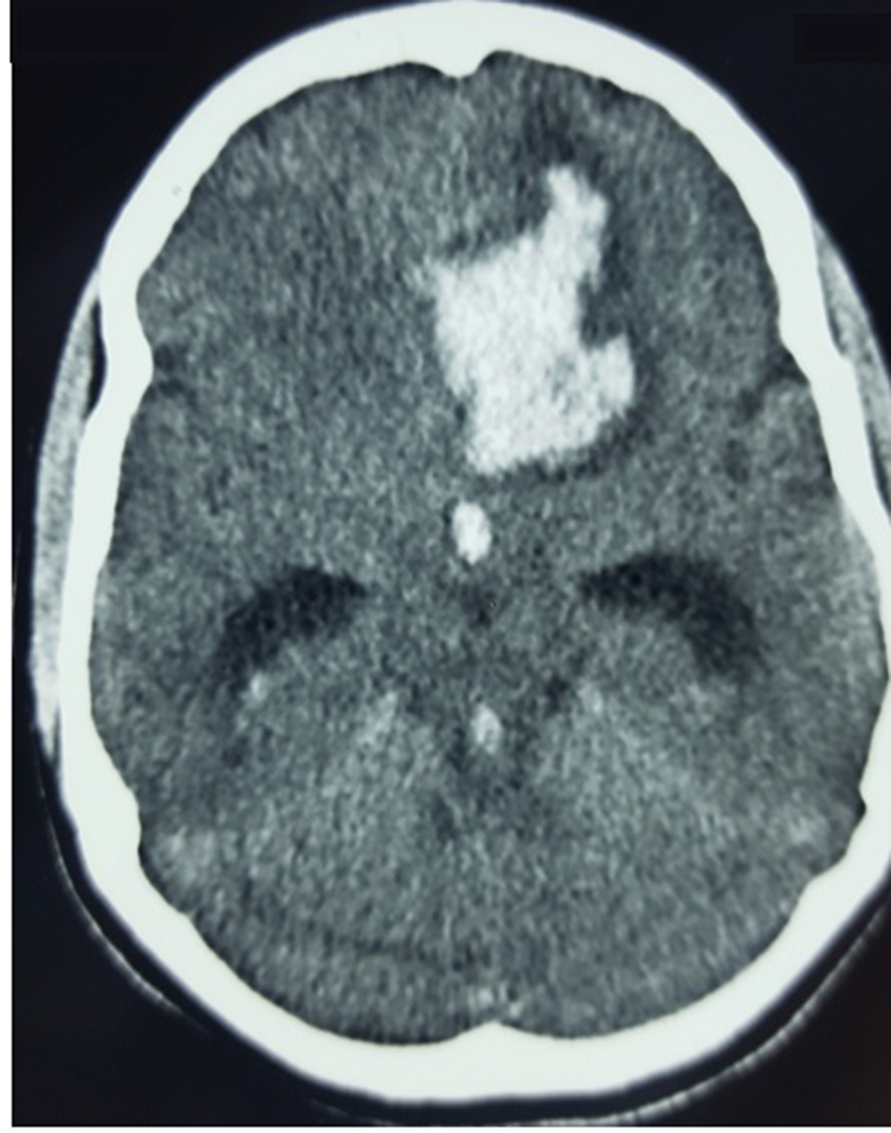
Axial CT scan after second bleed demonstrating left frontal haematoma opened to ventricular system with signs of acute hydrocephalus.
